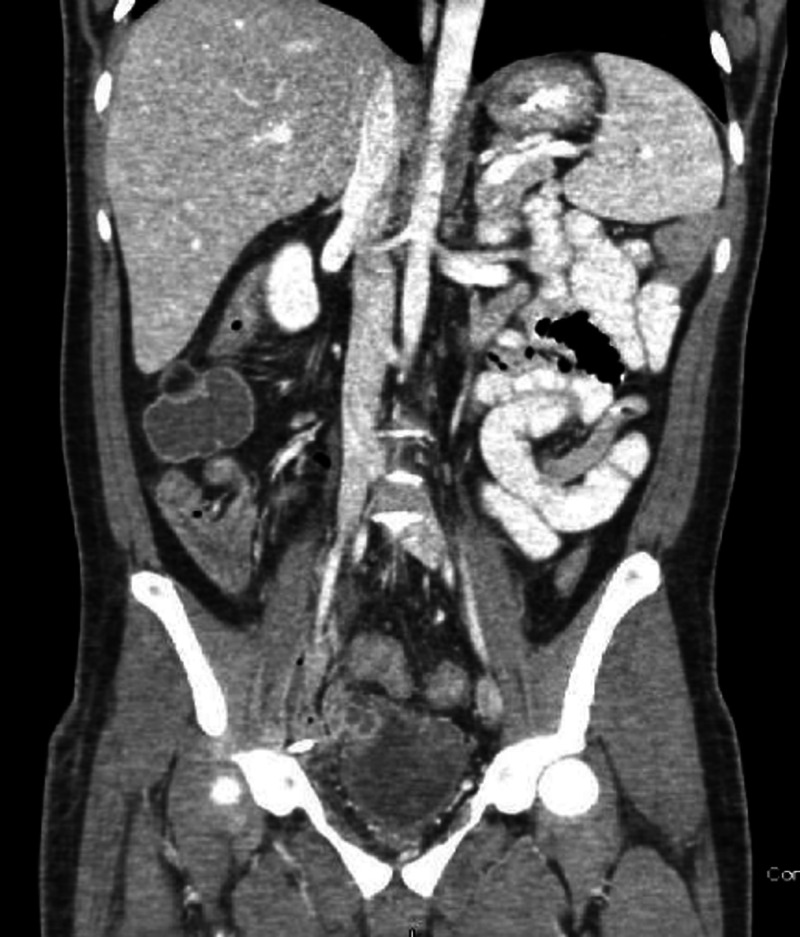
The 21-day postoperative CT scan (coronal section) showing abscess resolution. Drainage catheters have also been removed.
